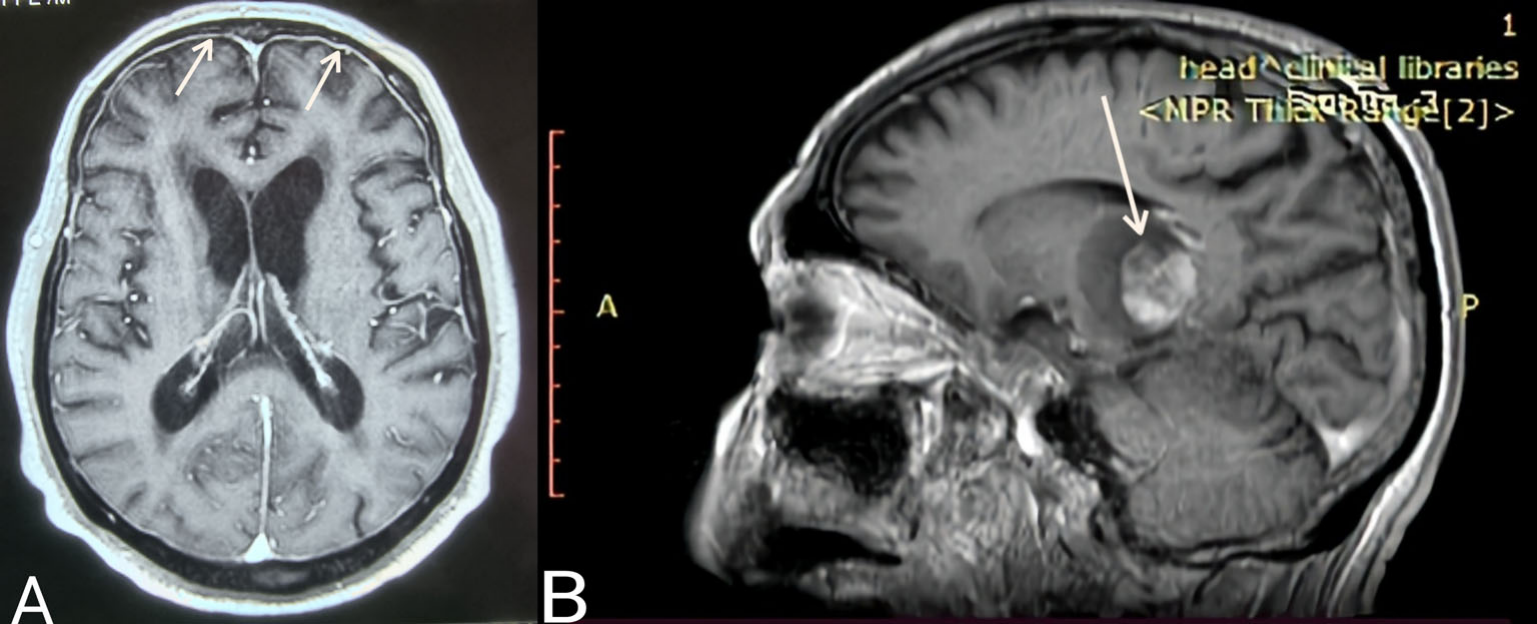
(A) Case 3: Axial T1 MR image (gadolinium enhanced) showing meningeal enhancement-diffuse form of BNS (white arrows). (B) Case 4: Sagittal T1 MR image (gadolinium enhanced) showing a brain mass- tumoral form of BNS (white arrow).
